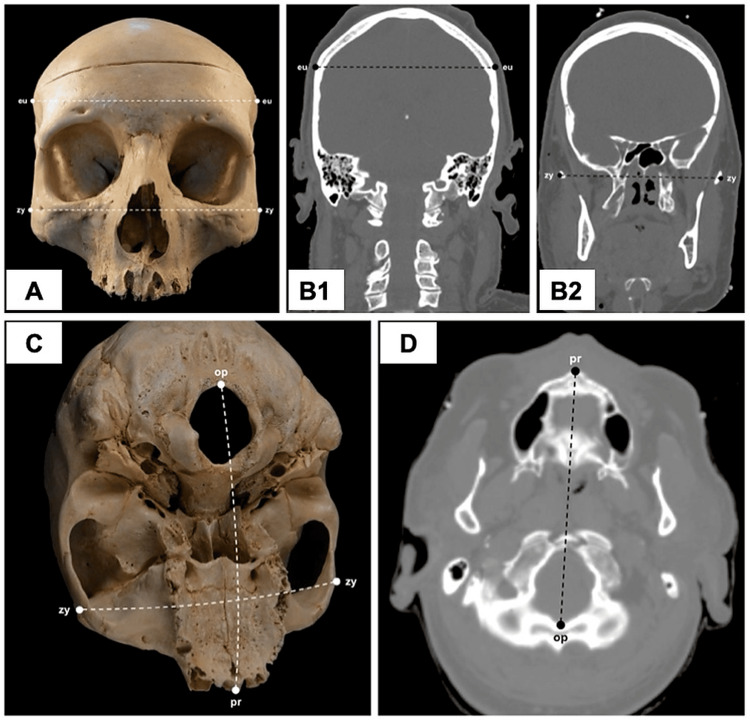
Craniometric variables assessed in dry skulls and CT examinations (A) Frontal dry-skull view showing maximum cranial breadth and bizygomatic breadth. (B1) Coronal CT image showing maximum cranial breadth. (B2) Coronal CT image showing bizygomatic breadth. (C) Inferior dry-skull view showing skull base length and bizygomatic breadth. (D) CT depiction of skull base length. CT: computed tomography; eu: euryon; op: opisthion; pr: prosthion; zy: zygion.
